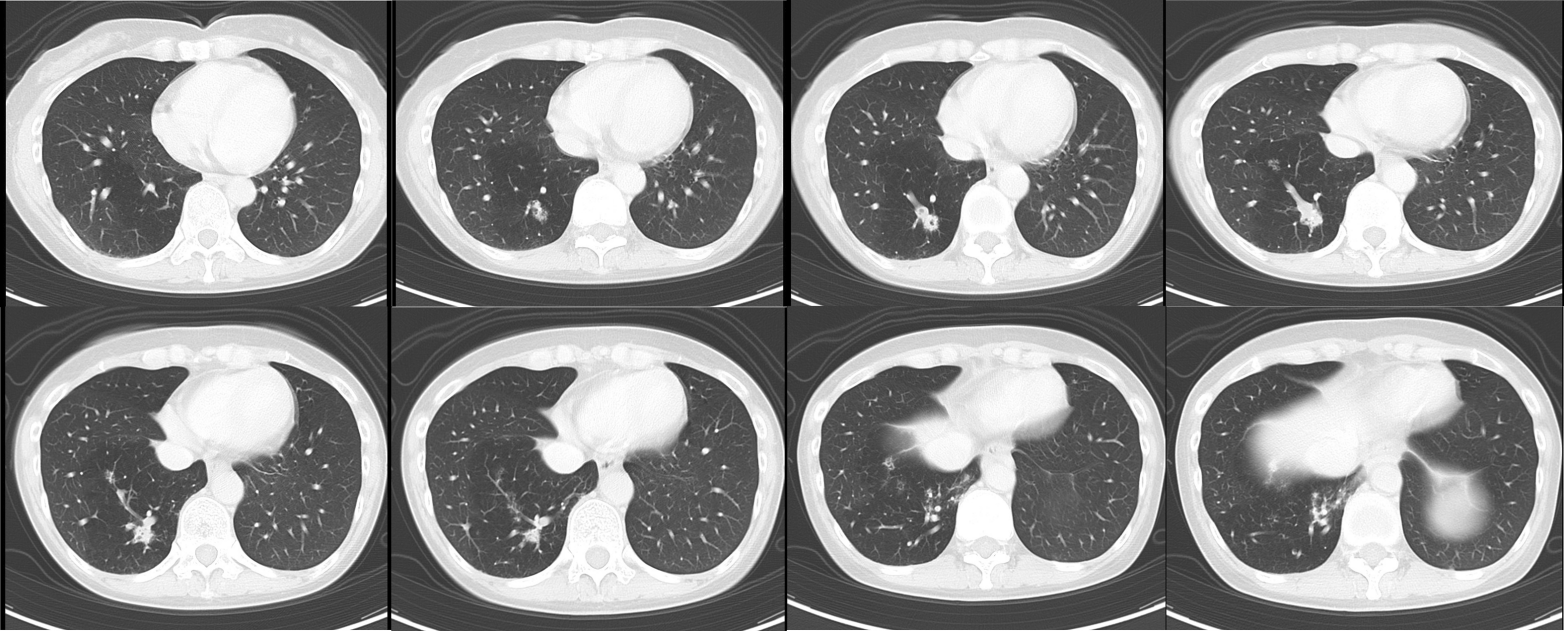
CE‐CT demonstrating multiple, nodular, pulmonary lesions with calcification in the posterior basal segment of the right lower pulmonary lobe with surrounding, focal emphysema.
